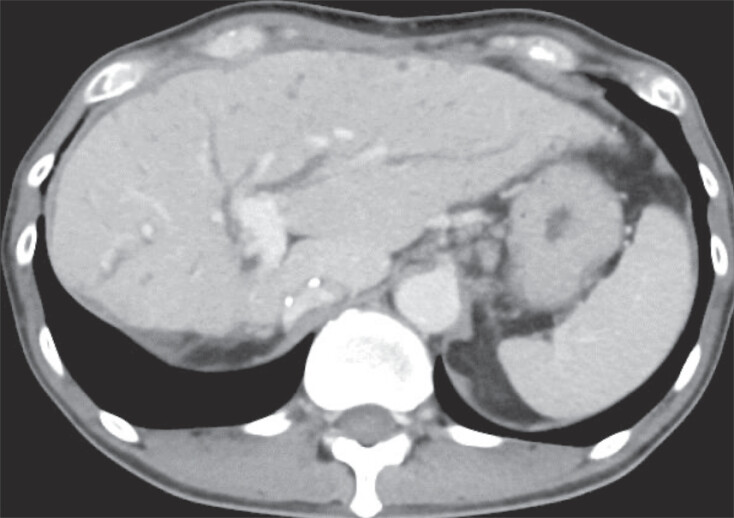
A computed tomography image shows dilated intrahepatic bile
